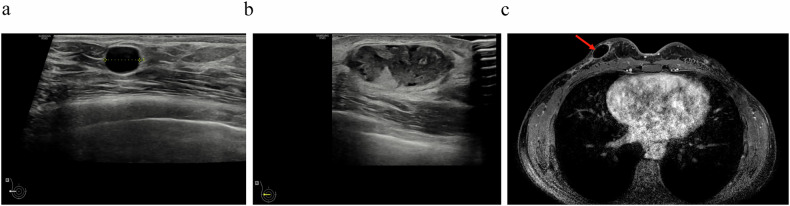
Fat necrosis after total AFT breast reconstruction. A 37-year-old patient with a history of bilateral prophylactic mastectomy, because of PALB2 gene mutation, underwent delayed total reconstruction of both breasts with AFT. After three AFT sessions, the patient presented with a painful lump in the right breast. Three palpable lumps were found during physical examination. Further diagnostics included US and MRI examination, which showed fat necrosis to be the cause. On US, the fat necrosis was described as a round mass, with mixed solid and cystic components. This was classified as BI-RADS 2 (a, b). MRI T1W-dynamic contrast-enhanced images showed a subcutaneous mass in the upper outer quadrant of her right breast, of 2.7 cm diameter with a hyperintense border (c; arrow)
